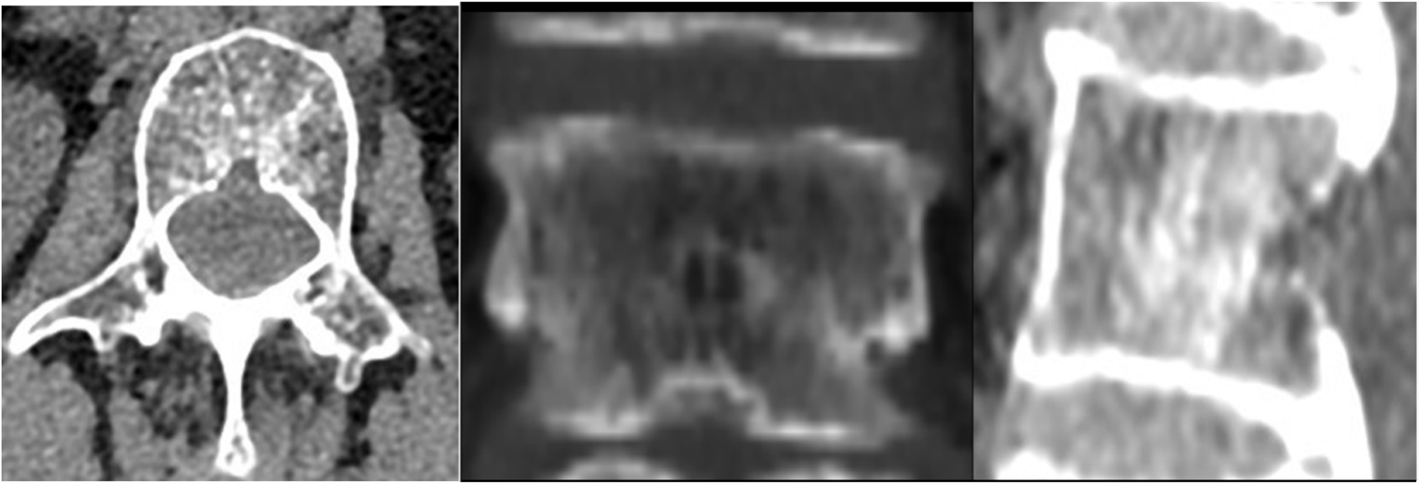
CT scan sagittal, coronal and water shape of vertebroid foramen of T12 vertebral body in patients with osteoporosis
